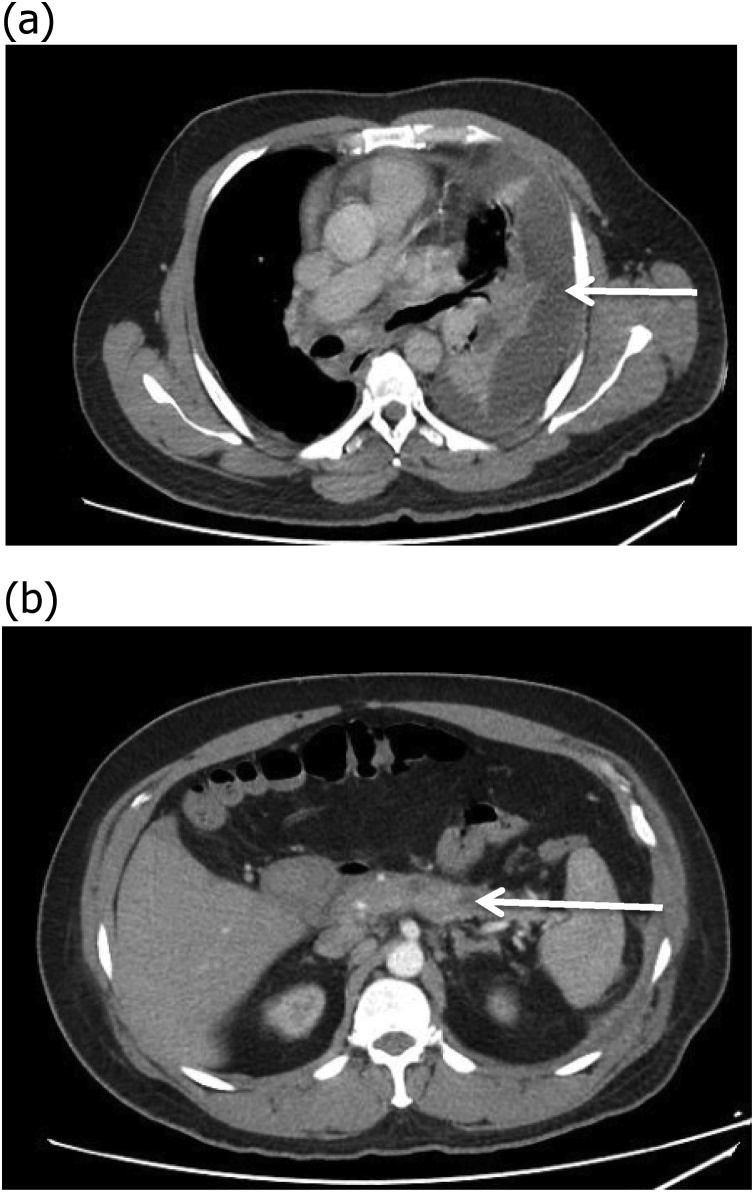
a. CT chest showing left sided loculated effusion. b. Diffuse thickening along the pancreas with atrophy of tail of pancreas and prominent pancreatic duct likely representing changes of chronic pancreatitis.
